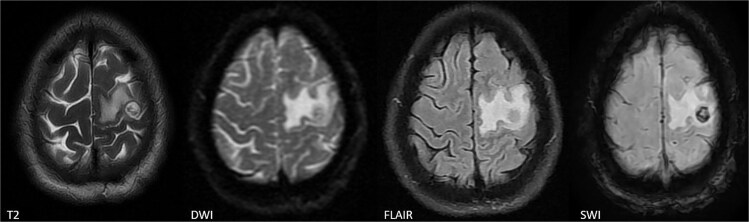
MRI sequences highly suggestive of a neurocysticercosis involving the left hand knob region.
